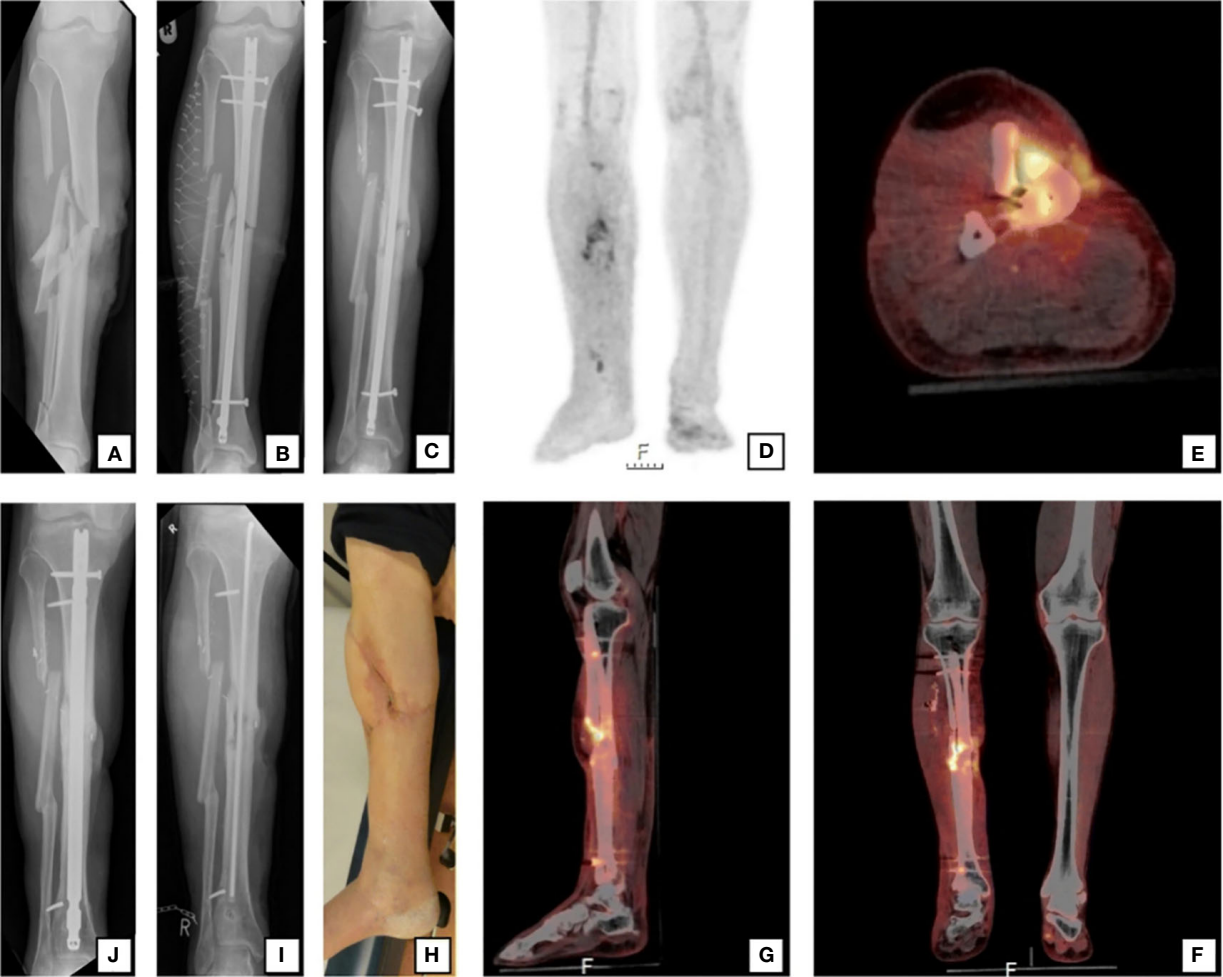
A 59-year-old man sustained a right-sided open crural fracture (A) which was treated with intramedullary nailing and a fasciotomy (B). After several soft-tissue debridement procedures, the remaining soft tissue defect was eventually closed with a free musculocutaneous flap. After 20 months, there was a non-union with “autodynamization” of the intramedullary nail, demonstrated by broken interlocking screws (C). The ^18^F-FDG PET/CT image (D) shows increased uptake around the fracture site in the tibial shaft and around the proximal and distal screws. The hybrid ^18^F-FDG PET/CT (E axial, F coronal, G sagittal) localize the suspected fracture-related infection (FRI) not only to the fracture site but also to the surrounding bone of the tibia around the fracture site which corresponds to the unstable scar overlapping the area of the non-union (H). The intramedullary nail was removed, the tibia was reamed, the fracture site was debrided and an in-house, custom-made antibiotic nail was inserted (I). FRI was confirmed by microbiological cultures and the patient was subsequently treated with antibiotics. One year after exchange nailing, fracture healing was successful (J)
